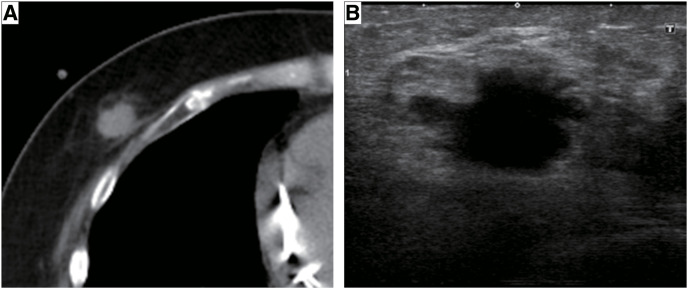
Imaging of breast cancer at diagnosis. (A) Plain computed tomography imaging showed right breast tumor. (B) Ultrasonography imaging showed hypoechoic 19 mm mass.
